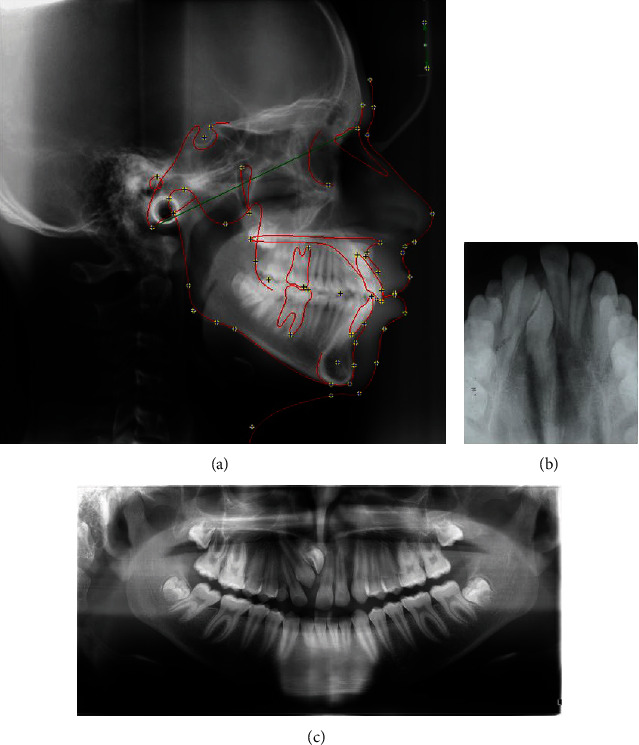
Pre-treatment radiographs. (a) Lateral cephalogram with tracing. (b) Occlusal radiograph. (c) orthopantomogram.
